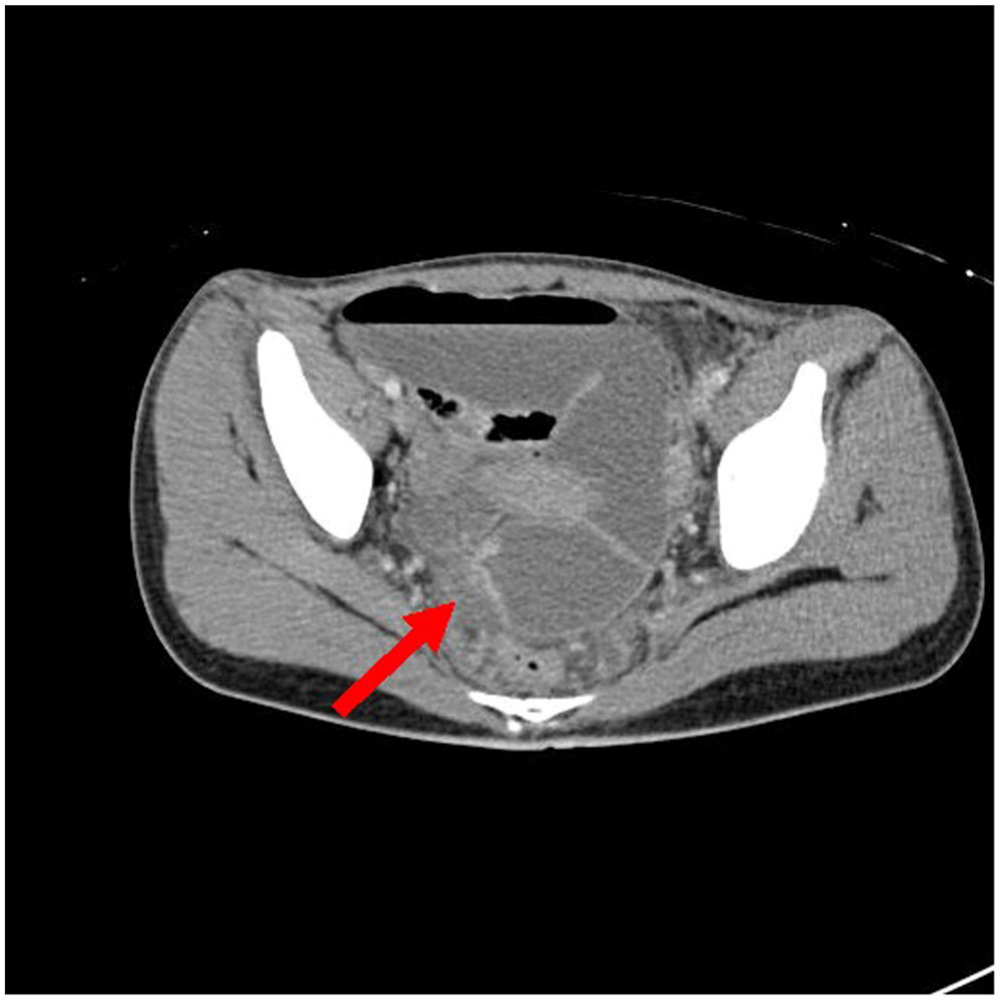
Computed tomography abdomen and pelvis with IV contrast. Axial image showing the distal ileal stricture as denoted by the arrow.
